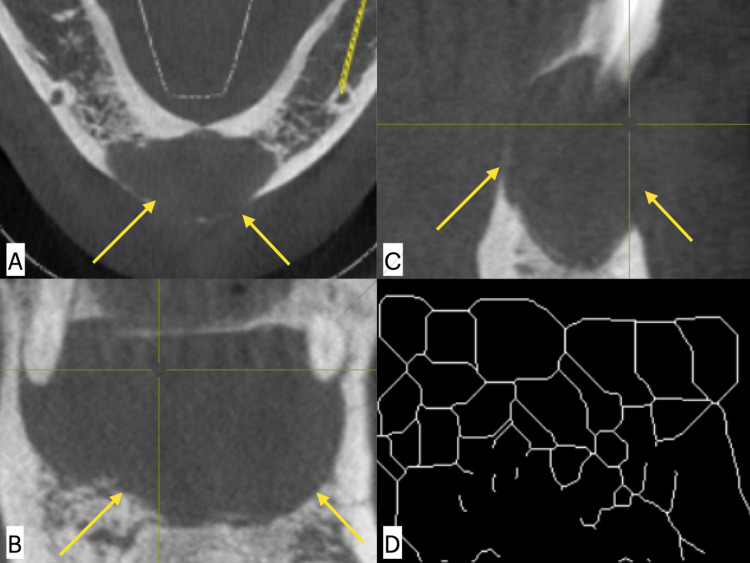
Radicular cyst (RC) in CBCT images measurement of the size of the lesion in (A) axial, (B) coronal, (C) sagittal planes, and (D) skeletonised fractal image. CBCT: cone beam computed tomography
